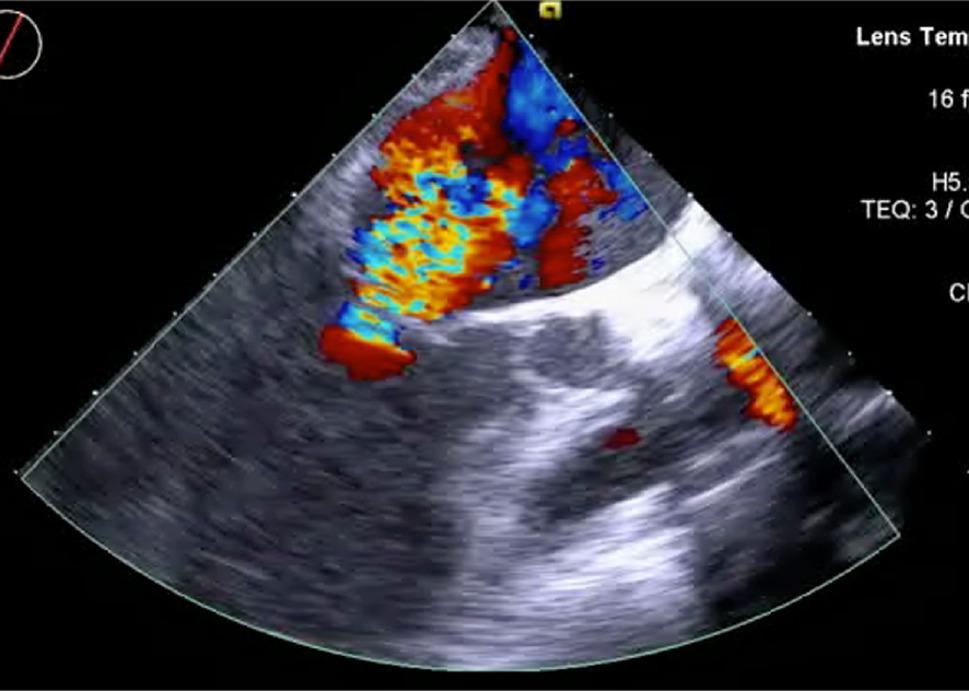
Intraoperative transesophageal echocardiography with color Doppler showing a posteriorly directed eccentric mitral regurgitant jet, suggestive of anterolateral papillary muscle dysfunction
